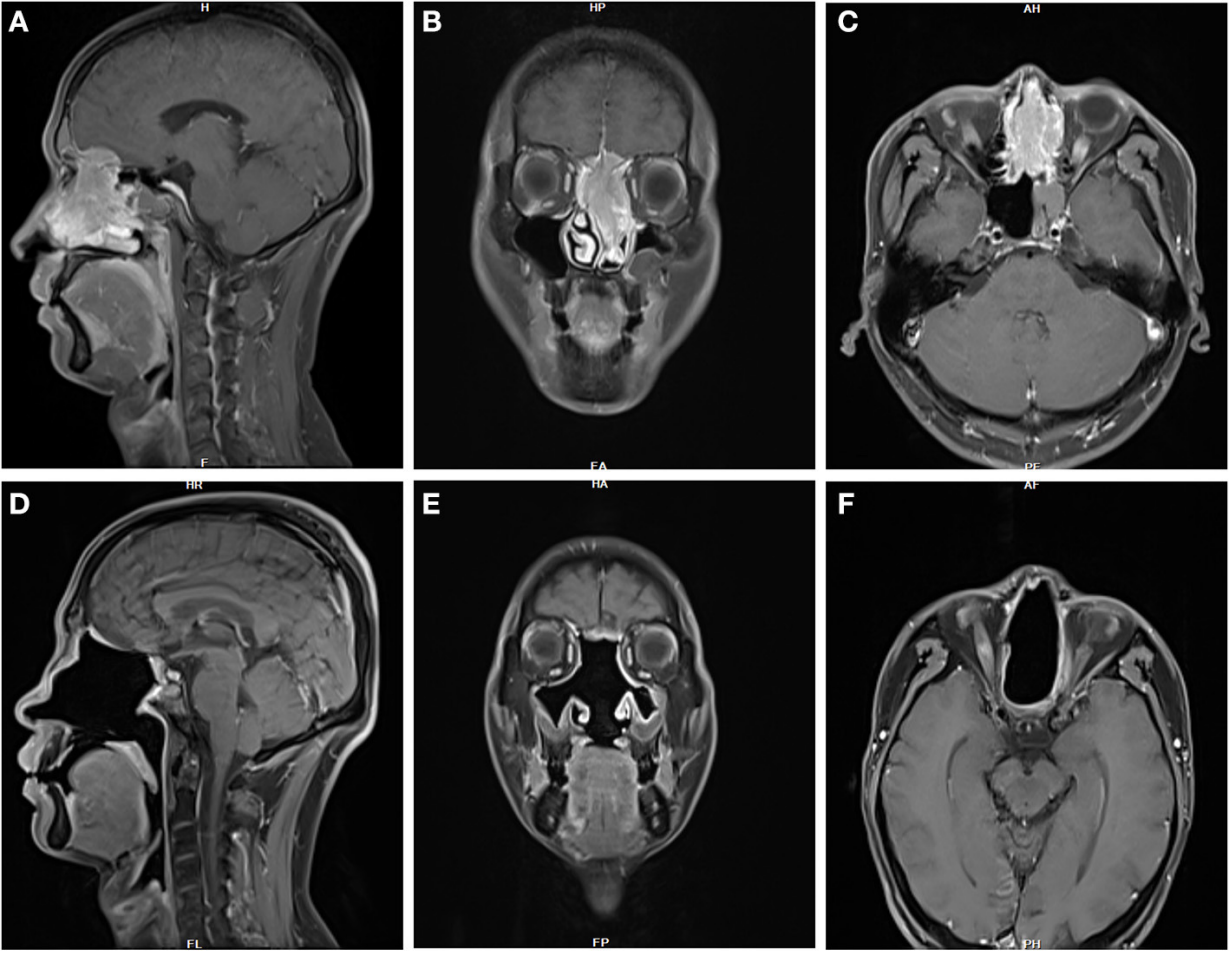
(A–C) MRI imaging in this 41-year-old male patient with persistent nasal obstruction revealed a large, left nasal mass. (D–F) The patient remained disease-free 29 months postoperatively.
